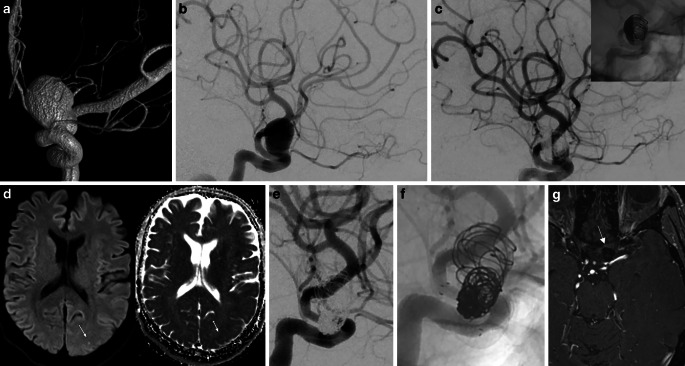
a 3D-angiography showing a large (maximum diameter 14 mm) saccular aneurysm in the paraophthalmic left ICA. b Subtracted DSA before endovascular treatment. c Subtracted and unsubtracted DSA after coiling and FRED implantation showing stasis in the aneurysm. d MRI scan after 24.9 months from the index procedure due to several episodes of amaurosis fugax while being under ASS monotherapy: DWI and ADC maps showing a punctate diffusion restriction (arrows) restriction in the left occipital lobe. e , f Subtracted and unsubtracted DSA of the same day showing the patency of the FD and adjacent vessels, very mild intimal hyperplasia, along with stable complete occlusion of the aneurysm. g MRI TOF sequence showing complete aneurysm occlusion at the 5‑year follow-up
